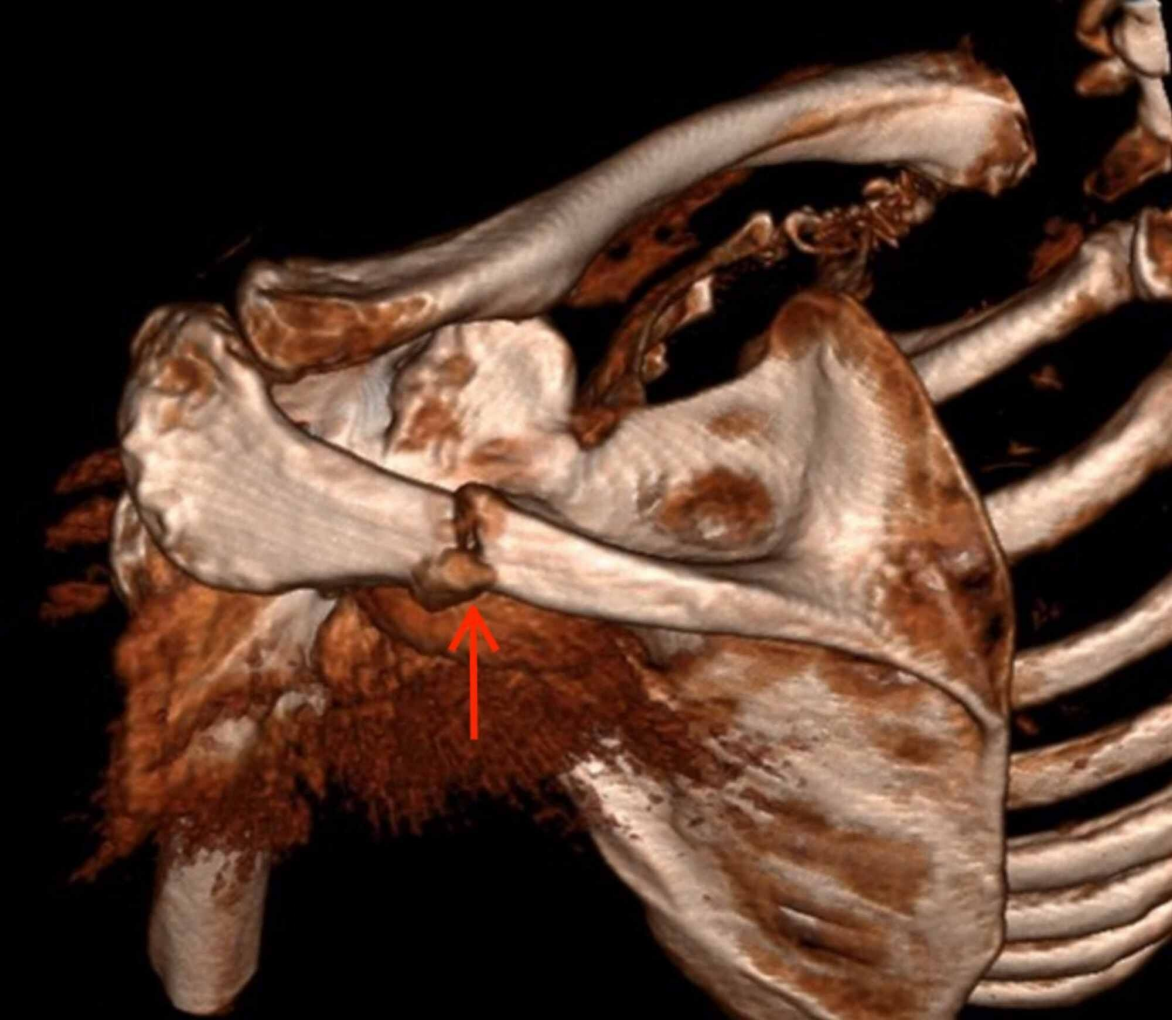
3D re-format CT scan demonstrated a fracture of the medial third of the left scapula spine CT: computed tomography
